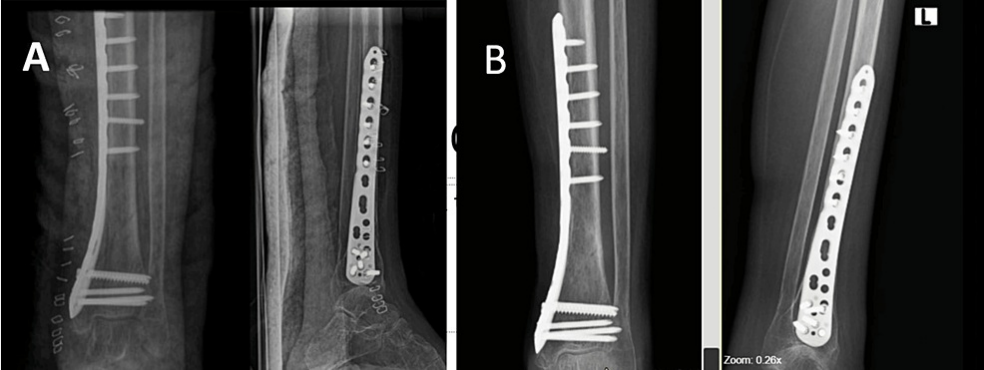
Postoperative radiographs. (A) Postoperative day one. (B) Six months post operation.
